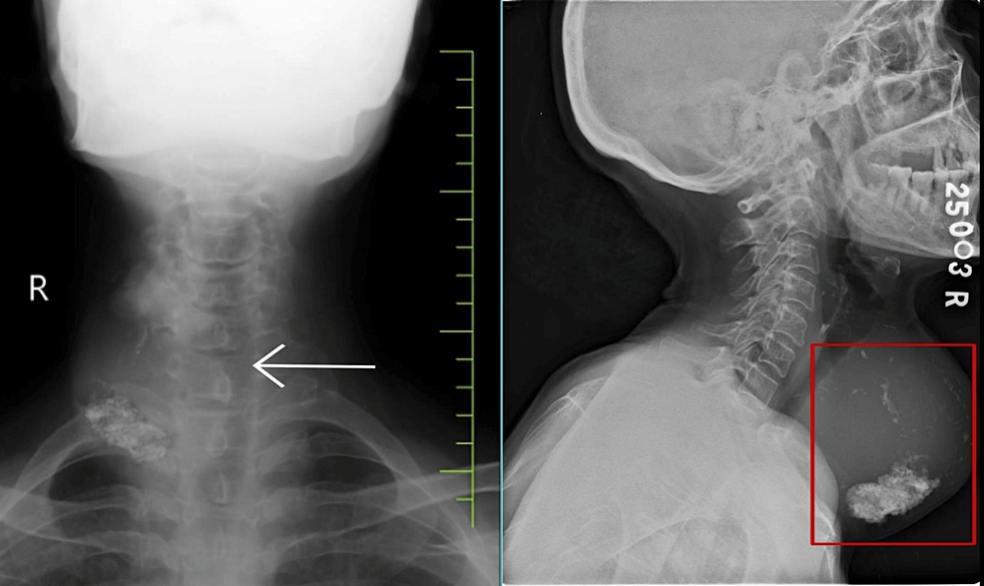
Neck radiograph in anterior-posterior view (left) and lateral view (right) White arrow showing a tracheal shift toward the left side. Neck swelling in the lateral view (red box) with evidence of macro calcification within
